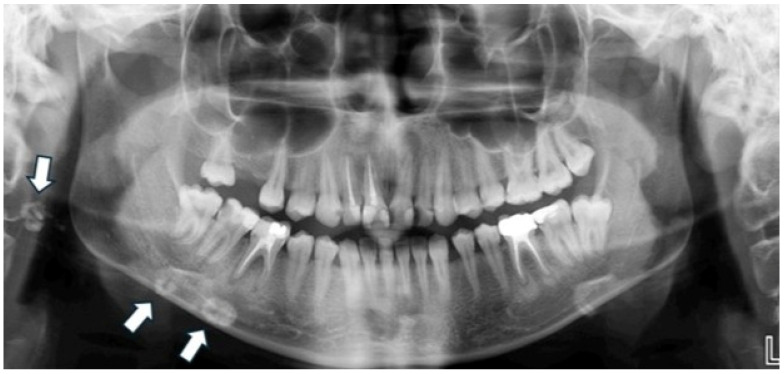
Panoramic radiograph of a 20-year-old female patient showing a unilateral, irregularly shaped radiopaque mass in the right submandibular region, suggestive of a calcified lymph node.
